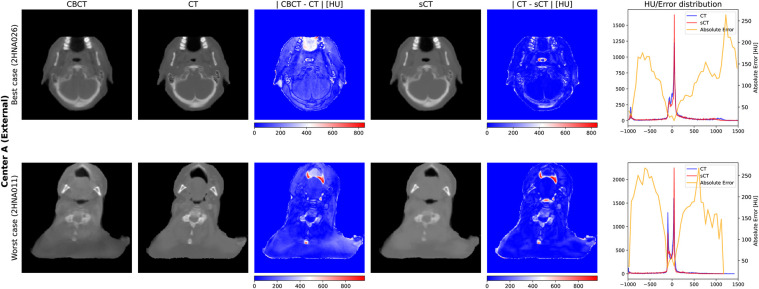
Visual evaluation of the best and worst sCT cases from Center A, used exclusively as an external test set. For each case, the mid-axial slice of the original CBCT, the ground-truth CT, the generated sCT, and the absolute difference maps for CBCT-to-CT and sCT-to-CT (error map) are shown. Additionally, intensity distributions of the sCT and CT for the displayed slice are presented, overlaid with the pixel-wise MAE. These distributions highlight the conversion accuracy across different tissue density ranges. Notably, regions with very high absolute differences appear in both CBCT-to-CT and error maps, suggesting the presence of residual misregistration between CBCT and CT scans.
